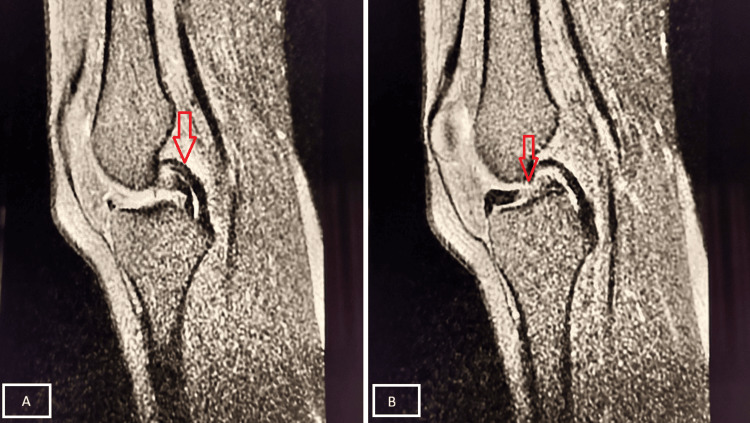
Bucket-handle tear of MM Sagittal PD-FS MRI shows a displaced fragment of the MM anterior to the PCL, producing the “double PCL sign” (A, red arrow). This finding represents a bucket-handle tear (B, red arrow) of the MM (Stoller Grade III meniscal tear). MM, medial meniscus; PD-FS, proton density fat-suppressed
